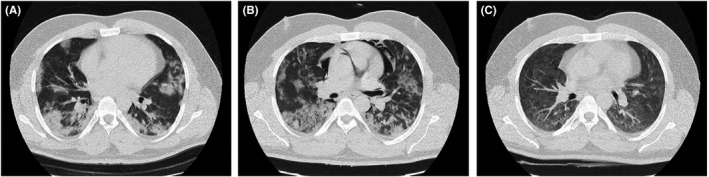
Chest CT scans of case 5
